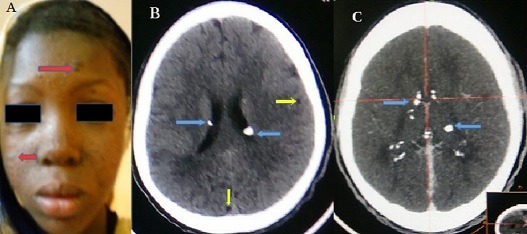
(Red) angiofibromas of the face (A); Brain CT Scan without injection of iodinated contrast agent (B) showing in axial sections calcified subependymal nodules (blue arrow) with supratentorial seat with bilateral asymmetric involvement and uncalcified cortical tubercles yellow arrow) C) Brain CT Scan with injection of iodinated contrast agent
